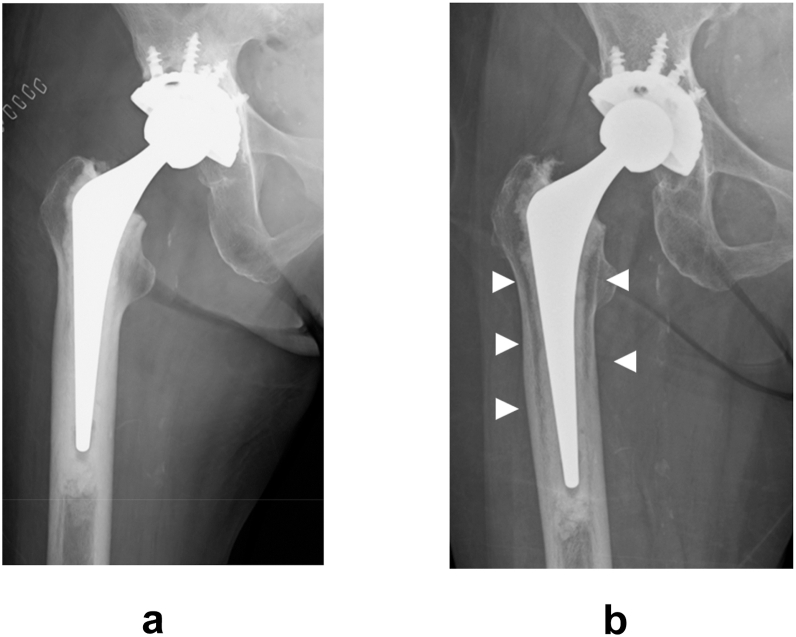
(a) Immediate postoperative plain radiographs, (b) plain radiographs at the final follow-up. The white triangular arrows indicate cancellisation.
